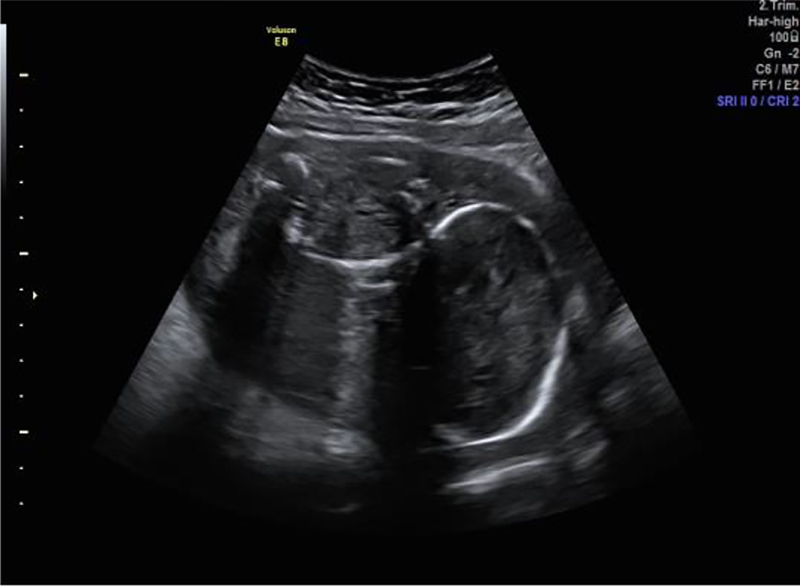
Ultrasound at 27 weeks showing a stopped foetal growth due to chronic anhydramnios.
